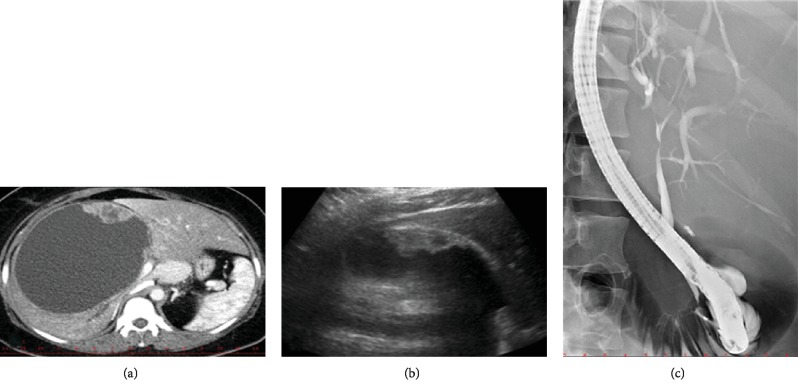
Imaging findings in a patient with biliary mucinous cystic neoplasm. (a) CT image of a large BMCN in the right liver lobe with a hypodense solid component. (b) Ultrasound image of the same BMCN with a hypoechoic solid component by the wall. (c) ERCP image of the same patient showing s smooth stricture of the hepatic duct caused by extrinsic compression of the cyst.
